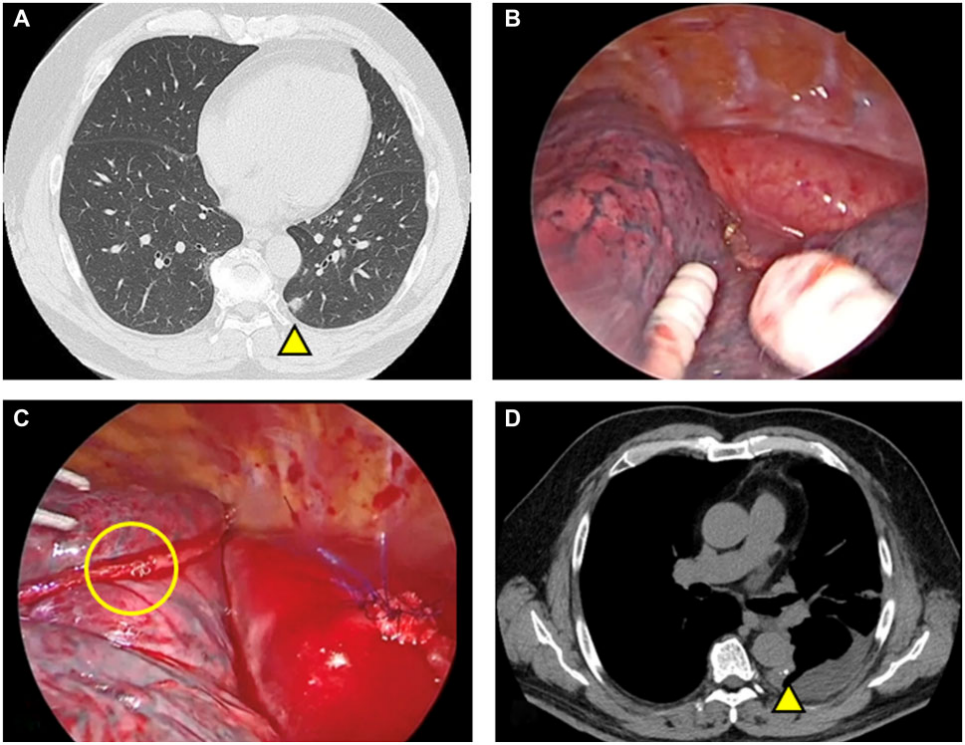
Preoperative and postoperative chest computed tomography and intraoperative findings. (A) Computed tomography revealed a lung nodule in the lower lobe of the left lung (yellow triangle). (B) Intraoperative findings of the first operation: No instrument-related injury to the aorta was found. (C) Intraoperative findings from the second operation comprised a wedge resection and the sutured aorta. The formation of an incomplete beta loop and damaged staples were not found on the stapling line, though a few staples on the stapling line were intertwined. (D) Postoperative chest computed tomography: The staple line contacts the aorta longitudinally.
